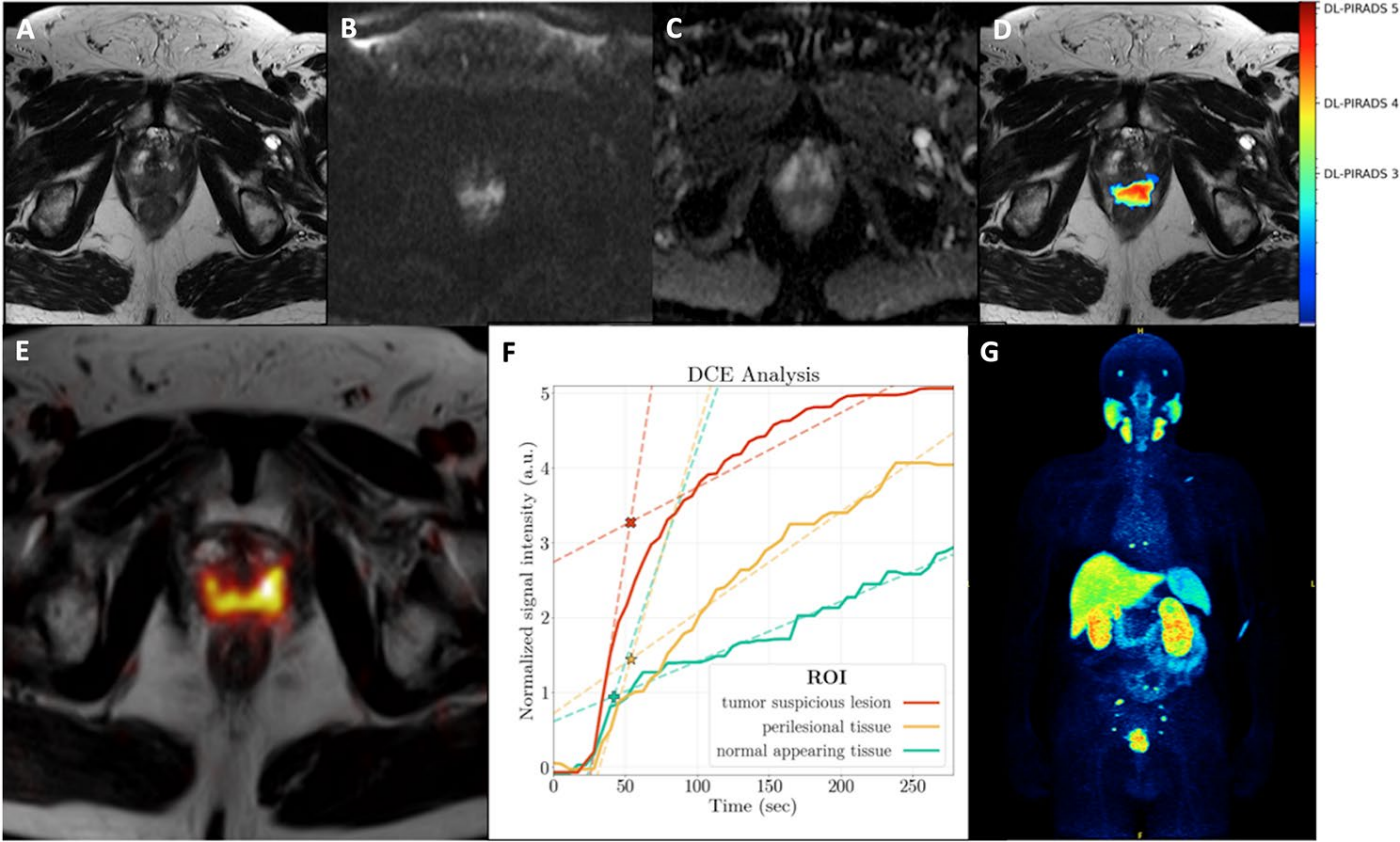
A 70-year-old patient with an initial diagnosis of PC (Gleason score 9) referred for initial staging with PET-MRI. His PSA level at the time of examination was 63.0 ng/ml. MRI showed a lesion suspicious for PC mainly in the posteromedial/-lateral peripheral zone with infiltration in the seminal vesicles (not shown) with T2w hypointensity (A) and diffusion restriction with increase in high b-value (B) and decrease in ADC map (C). The AI probability score is in good agreement with the MRI findings and also shows a high probability score for PC (DL-PIRADS 4, D). Quantitative data of DCE based on manual segmentations showed a steeper wash-in slope for tumor-suspicious lesion (TSL, red) compared to perilesional tissue (PLT, yellow) and normal appearing tissue (NAT, green). The corresponding fitted maximum (*, intersection of wash-in and wash-out slope) was 3.3 for TSL, 1.4 for PLT and 0.9 for NAT, enabling a clear distinction between TSL, PLT and NAT. All three curves showed an increasing curve pattern (F). Whole-body [^18^F]-PSMA-1007 PET imaging revealed the primary tumor as well as multiple PSMA-avid iliac lymph node metastases and osseous metastases in the sacral bone, thoracic spine and left scapula (G)
